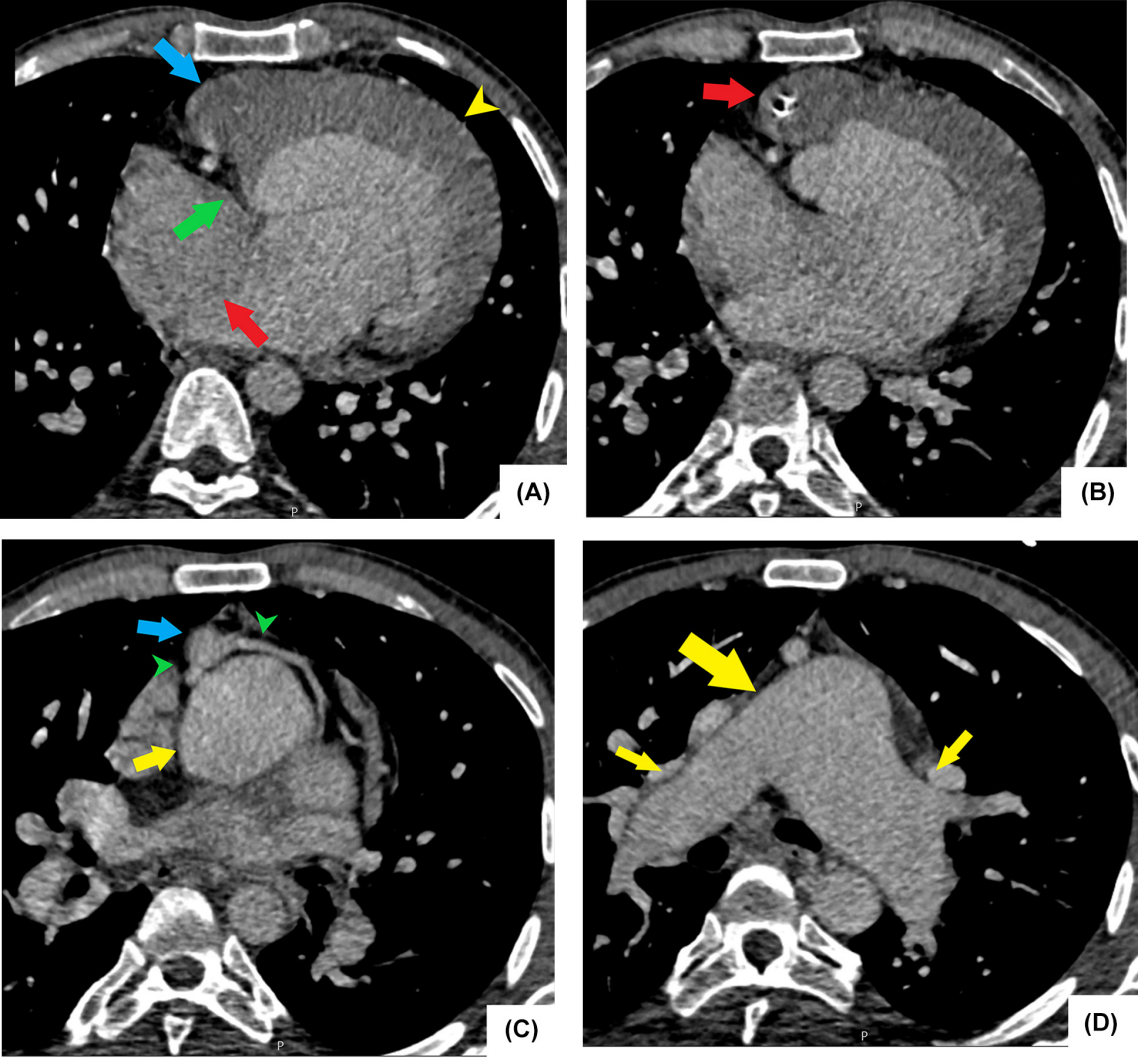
Cardiothoracic CT scan. Axial images show (A) four‐chamber image shows atresia of the tricuspid valve (green arrow) and hypoplastic right ventricle (blue arrow). There is a large atrial septal defect (red arrow), and the left ventricle is enlarged and hypertrophic (yellow arrowhead). (B) Atretic and calcified aortic valve (red arrow) originating from hypoplastic RV (L‐TGA). (C) Hypoplastic ascending aorta (blue arrow) located anterior to the main pulmonary artery (yellow arrow). Both coronary arteries (green arrows) have normal anatomy. (D) Dilated central pulmonary arteries (yellow arrows) indicate pulmonary hypertension.
